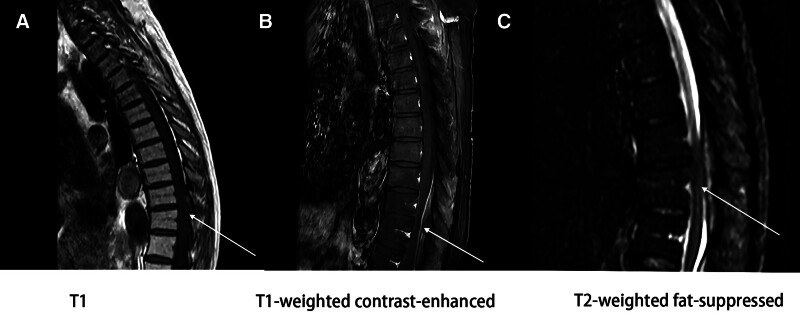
Enhanced MRI. (A–C) The area indicated by the arrow shows an abnormal signal in the epidural space at the posterior margin of the T10–12 vertebral canal. A thoracic spinal space-occupying lesion is considered. MRI = magnetic resonance imaging.
